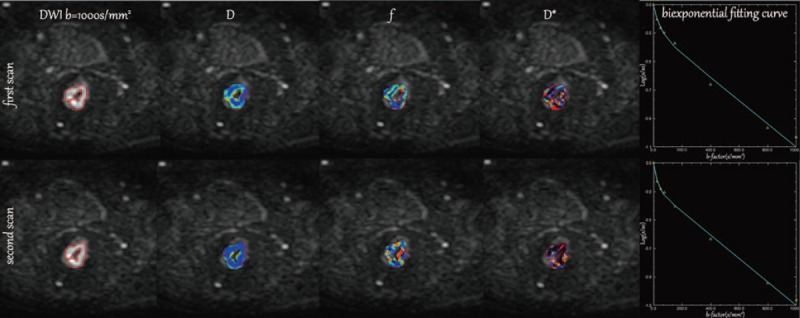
Twice-scanning images (including diffusion-weighted image [b = 1000 s/mm^2^], parametric IVIM color maps of rectal cancer [*D, f*, and *D∗*], plots of signal decay and biexponential fitting curves) of a 52-year-old male patient with middle-rectal cancer. Region of interests were manually drawn to contour the border of the rectal cancers on the slice (DWI images) with the maximum lesion size in twice-scanning. The upper line: the first examination images. The lower line: the second examination images.
